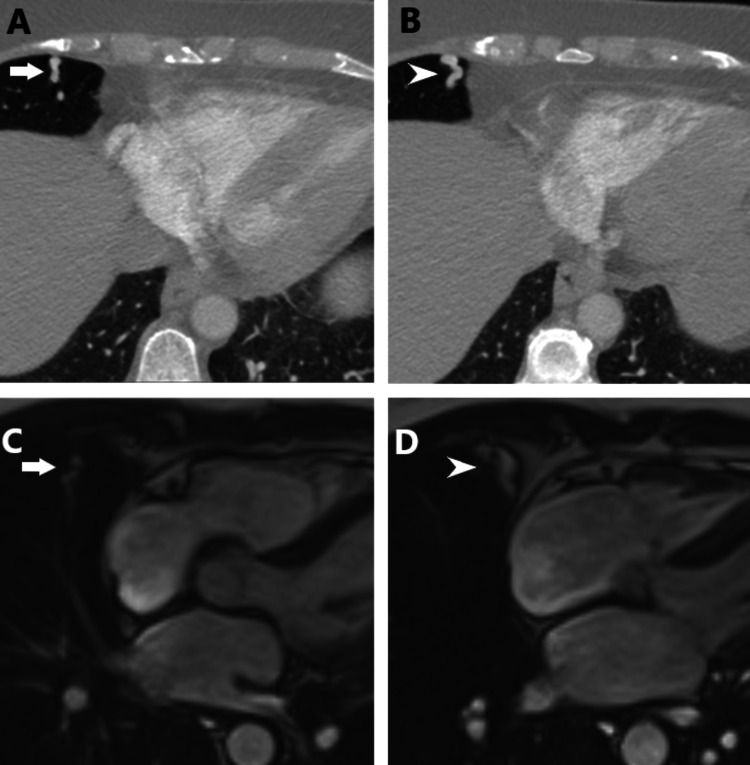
PAVM on CTPA and MRI Axial contrast-enhanced pulmonary angiography images (A, B) show the feeding artery site (white arrow in A) and draining vein site (white arrowhead in B) of a simple type of pulmonary arteriovenous malformation within the right middle lobe in a 53-year-old-woman. Corresponding steady-state free precession cine MRI images (C, D) show the feeding artery site (white arrow in C) and draining vein site (white arrowhead in D).
